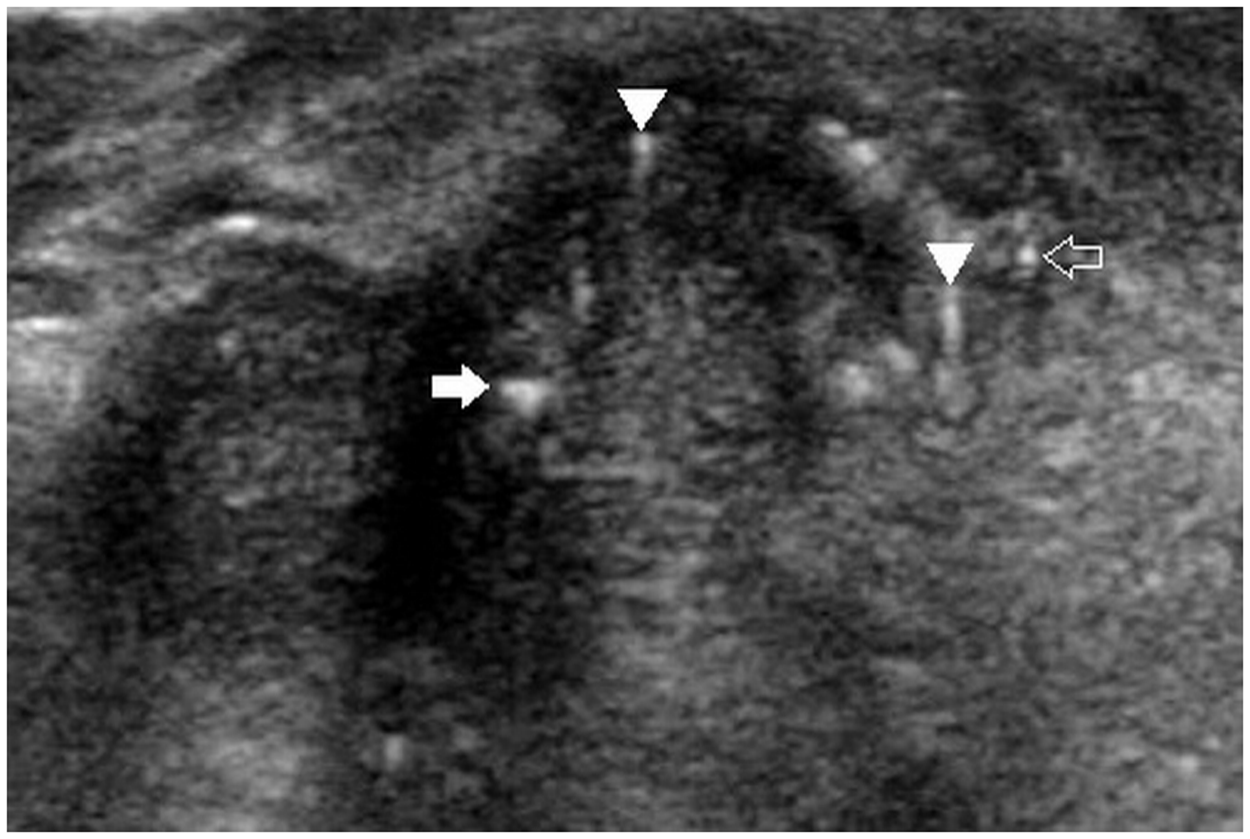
A 52-year-old woman with papillary thyroid carcinoma. Longitudinal sonogram showing a 45-mm, ill-defined, hypoechoic solid nodule in the right thyroid lobe. A linear echogenic focus with reverse-triangle artifact (solid arrow), and a round echogenic focus with fine artifact (arrowhead), and a punctate echogenic foci (faint arrow) are seen (Type 3).
